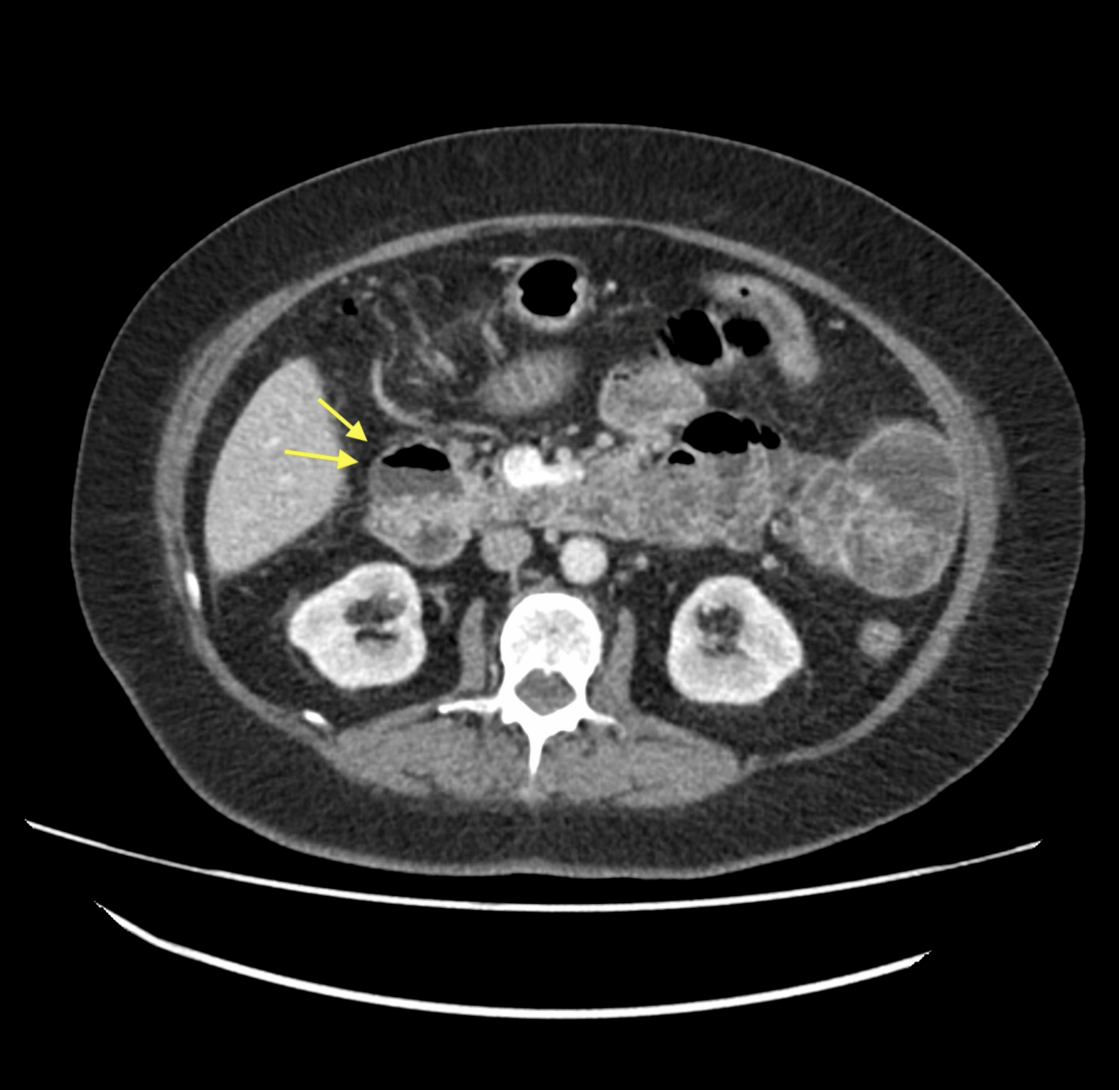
Axial CT slice shows an incidental finding of a duodenal diverticulum, arising from the second part of the duodenum CT: Computed tomography
